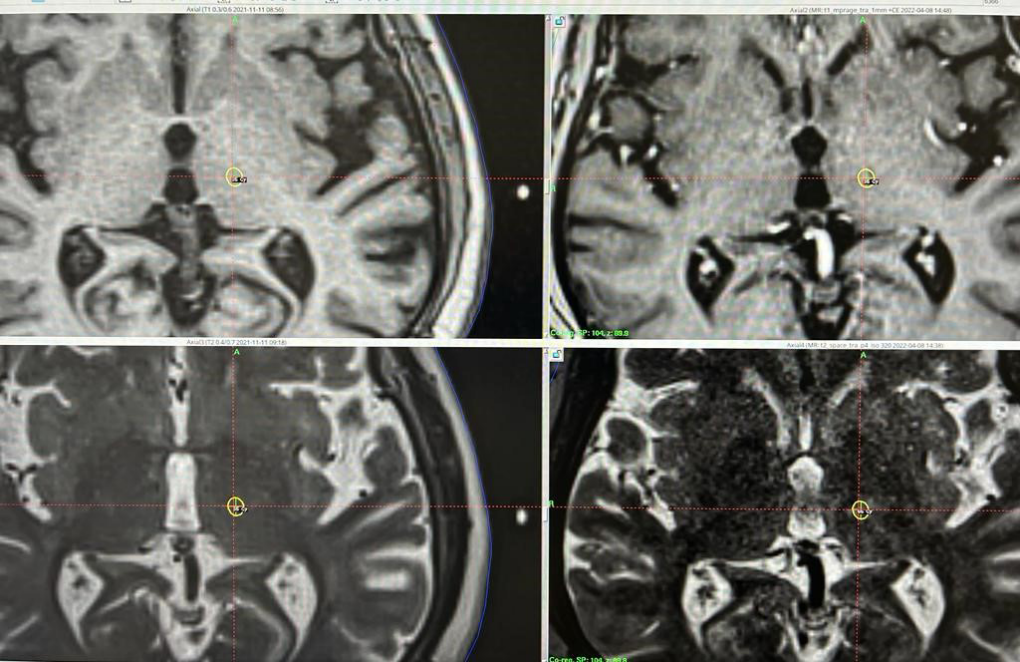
A brain magnetic resonance image (MRI) of case 2 comparing a baseline MRI (top and bottom left) to a control MRI (top and bottom right) done eight months after gamma knife thalamotomy. At the top are T1 and T1 magnetization-prepared rapid gradient echo sequences, and both sequences at the bottom are T2. An adequate irradiation lesion of the left thalamic ventral intermediate nucleus is presented.
